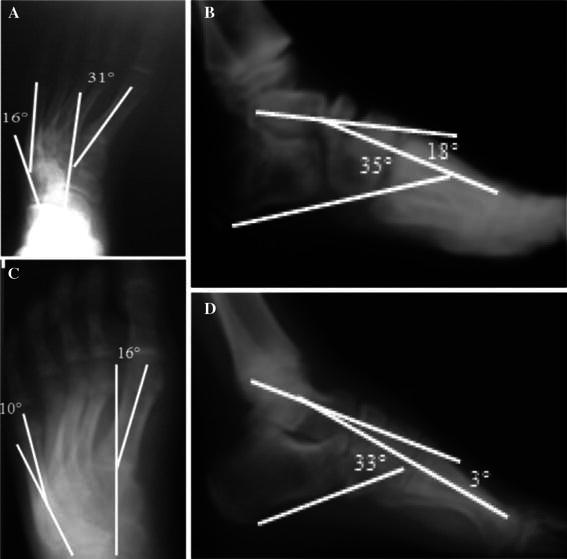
The preoperative anteroposterior (a) and lateral (b) radiographic view of the patient’s left foot is shown. The postoperative anteroposterior (c) and lateral (d) radiograph of the left foot is shown following this procedure, demonstrating correction of adduction in both views
